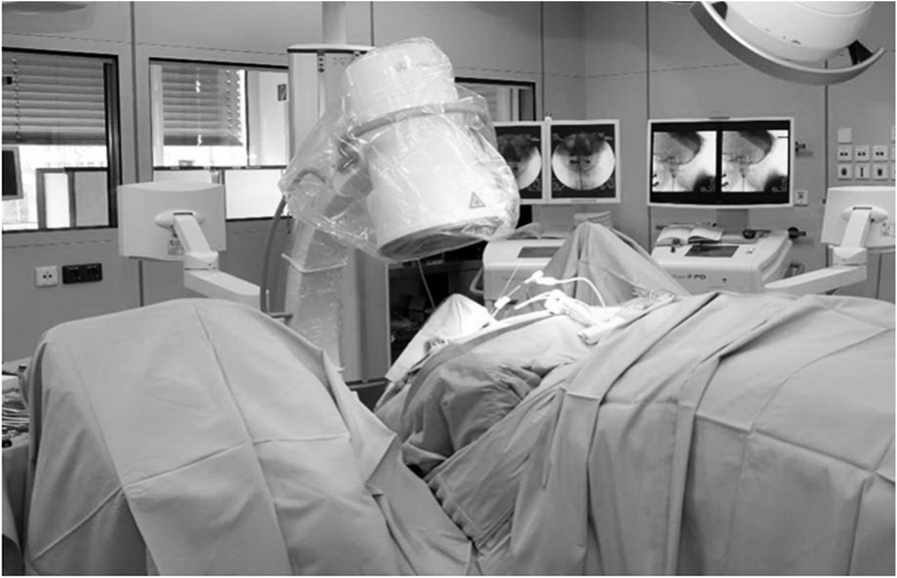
This picture shows the set-up in the OR with two c-arm X-ray units for anterior-posterior view, as well as for lateral view of the dens axis
